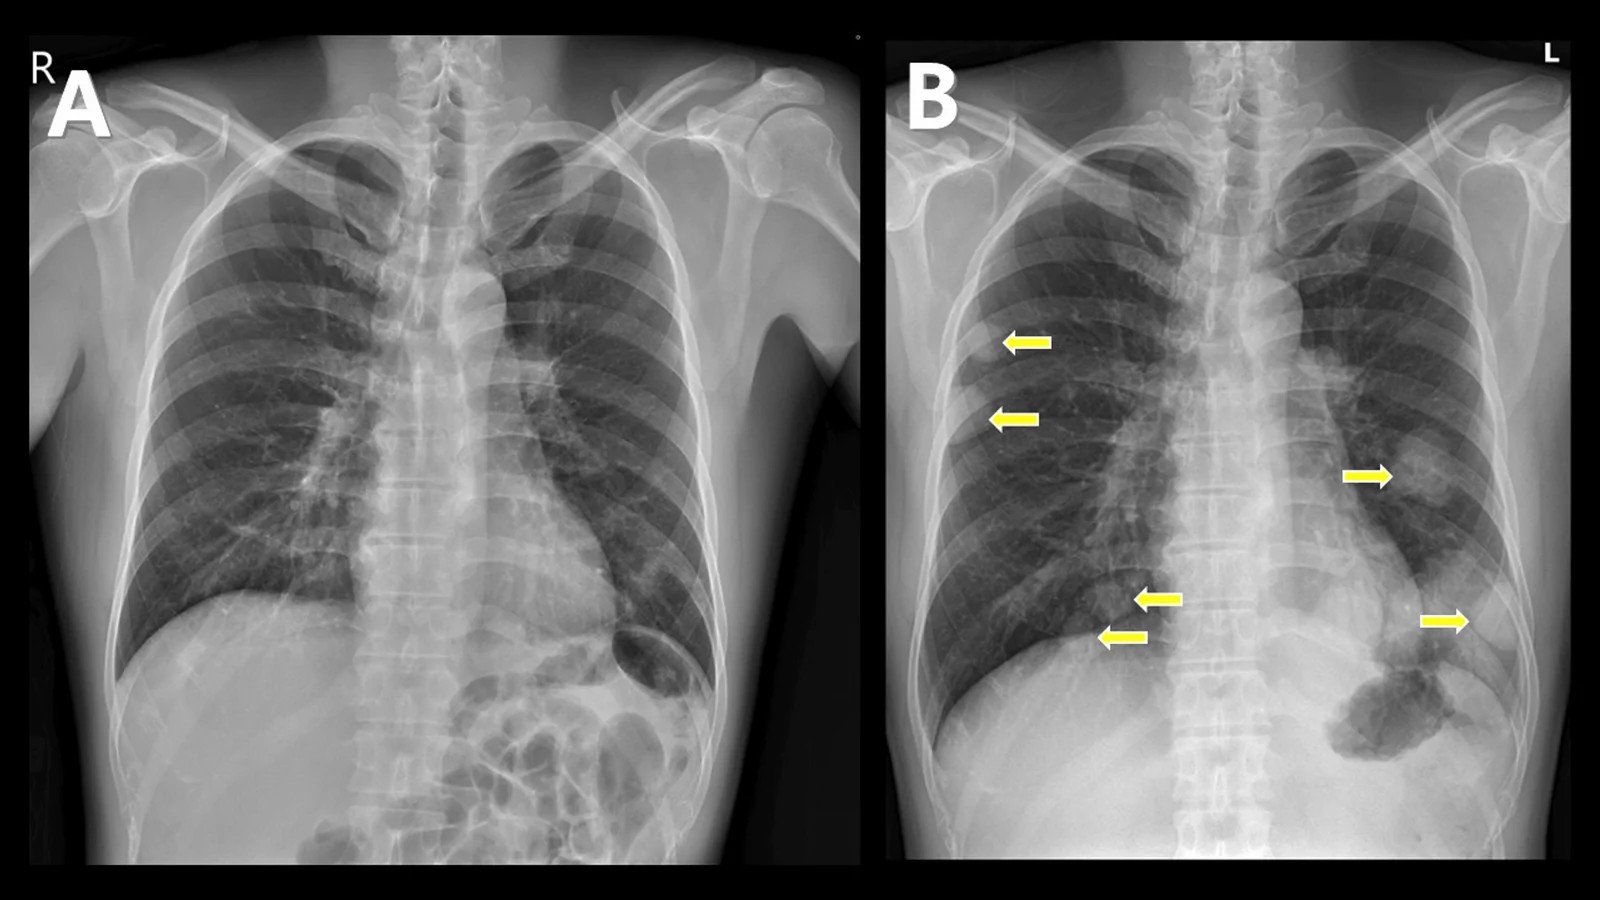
Initial and follow-up chest X-ray images (A) The previously performed chest X-ray shows no mass lesions. (B) The follow-up chest X-ray demonstrates multiple masses throughout both lung fields (arrows)
